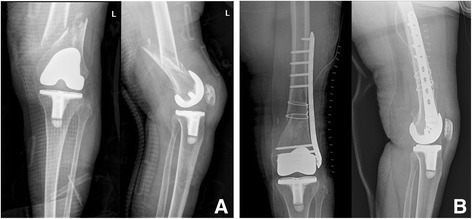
At 16 years after total knee arthroplasty (TKA), the patient visited our hospital for knee pain after a fall. a The radiograph shows a periprosthetic fracture around the prosthesis. b Postoperative radiograph taken after open reduction and internal fixation with a plate and wiring
